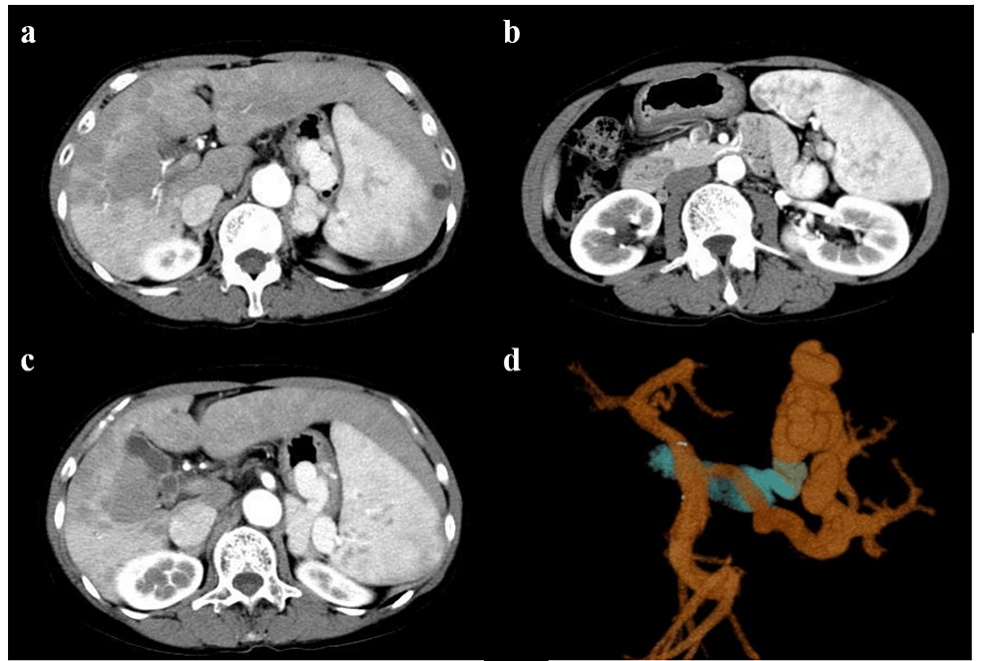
Abdominal contrast-enhanced computed tomography (CT) scan in the arterial phase a. Heterogeneous contrast effect is noted in the liver, the intrahepatic portal vein is narrowed, and a small mural thrombus is observed in some areas; b. There is marked splenomegaly and the splenic veins are dilated; c, d. Gastric varices are seen in the gastric vault. The short gastric vein is the main blood supply draining into the gastrorenal shunt.
